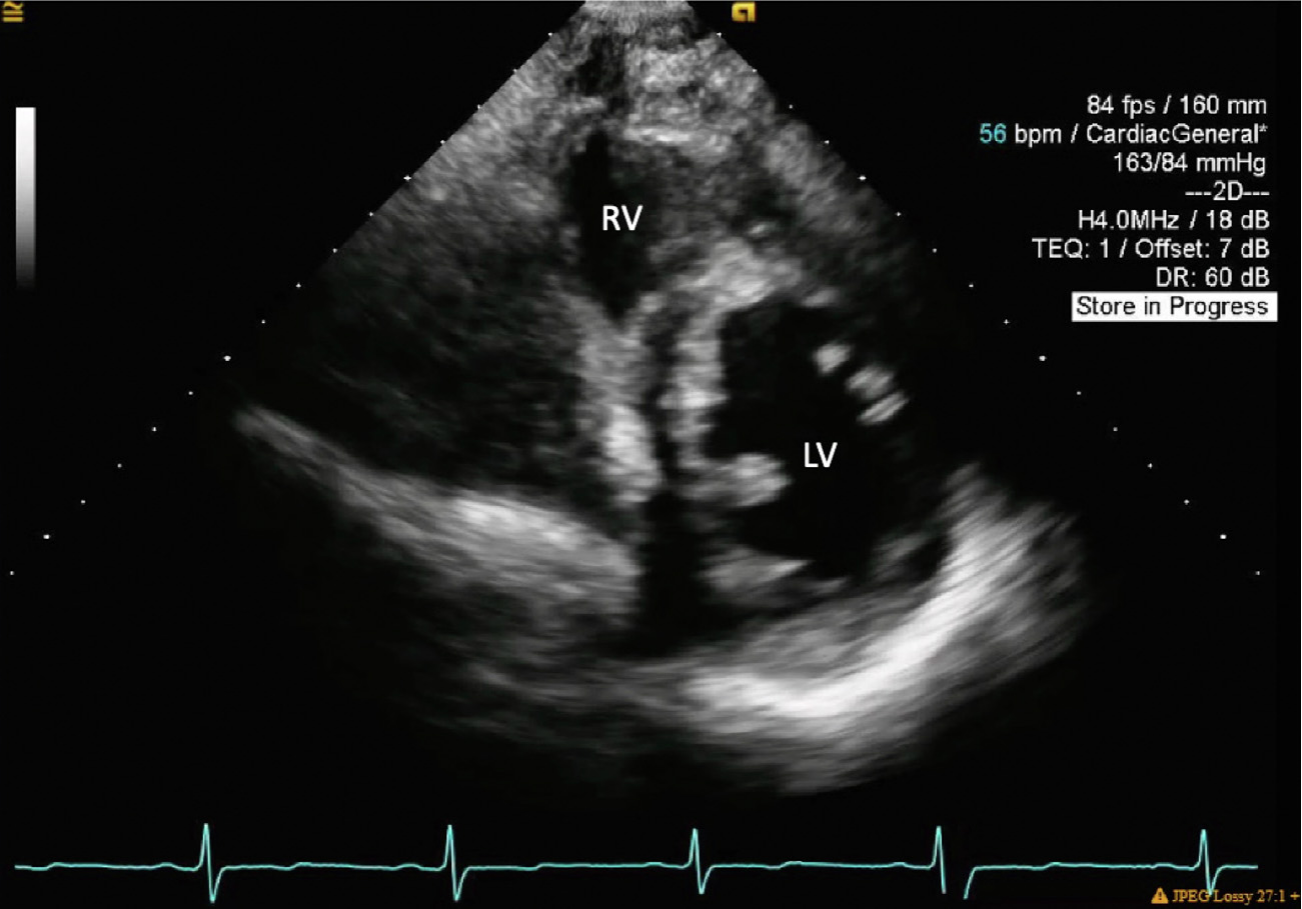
Two-dimensional TTE, parasternal short-axis view, midventricular, diastolic phase, demonstrates normal-appearing papillary muscles and conventional attachment of the papillary muscles to the chordae tendineae. *LV*, Left ventricle; *RV*, right ventricle.
